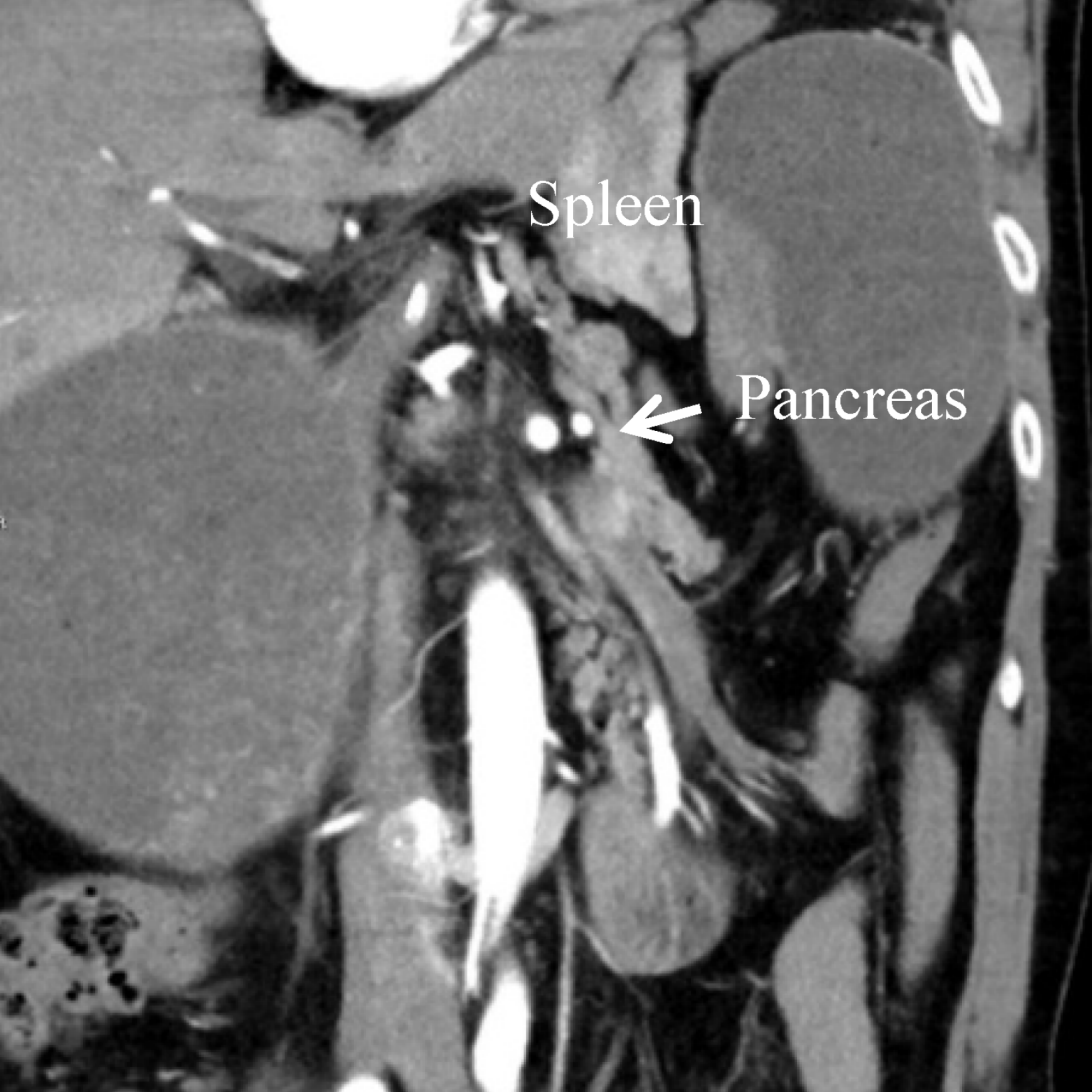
CT scan: spleen and pancreas tail shifted medially
